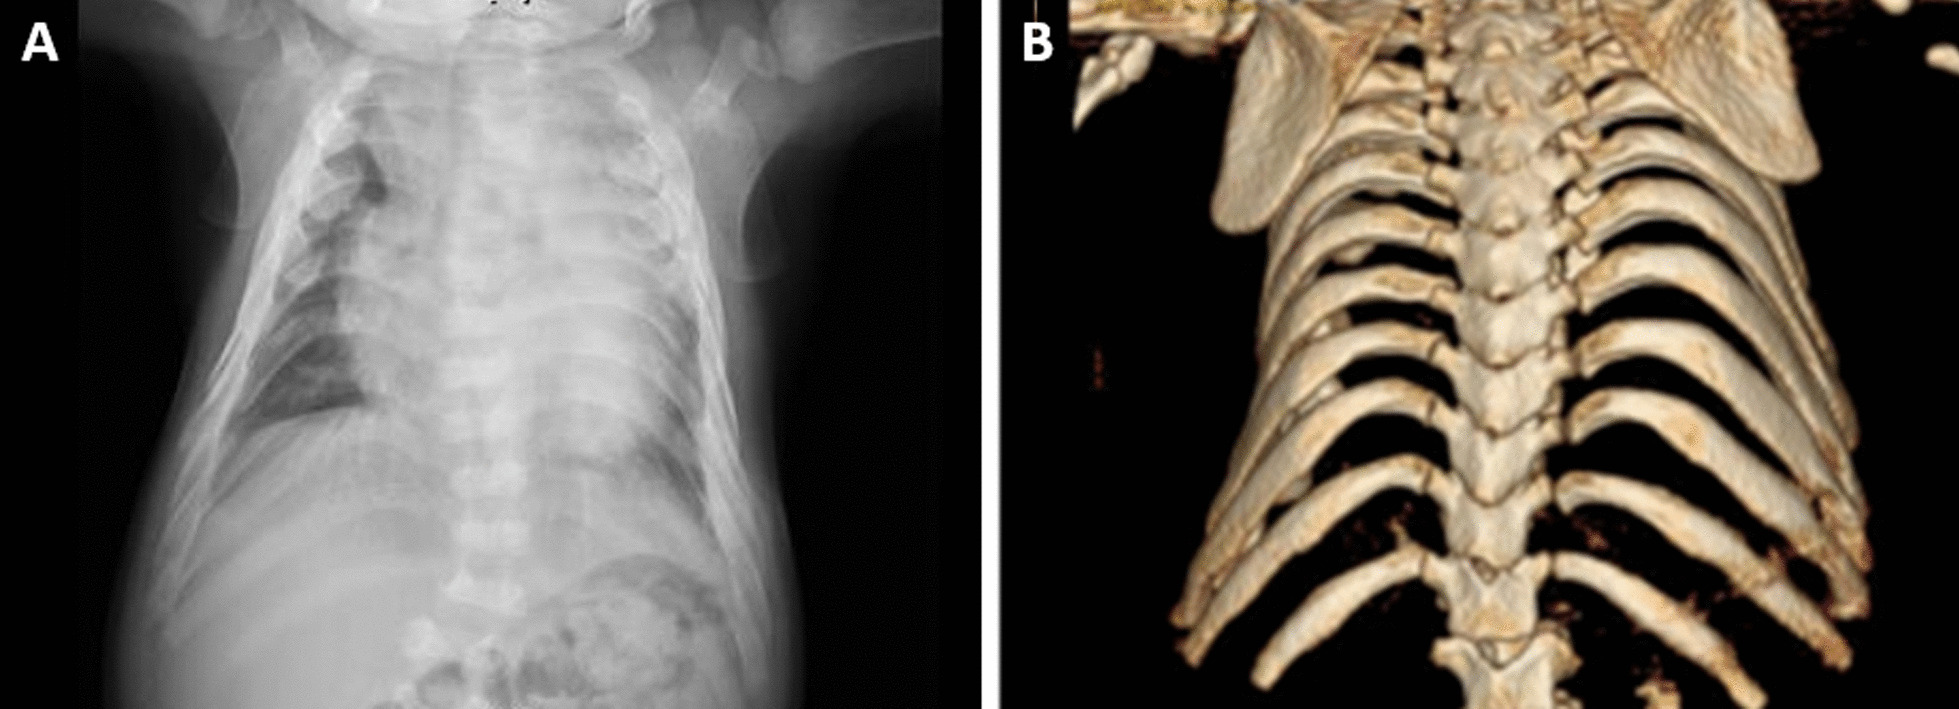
X-ray and MRI images of a MPS VII patient. a Marked cardiomegaly, and thoracic deformity causing a significant loss of volume of the left lung parenchyma. b Small vertebral bodies, with hook-like morphology
